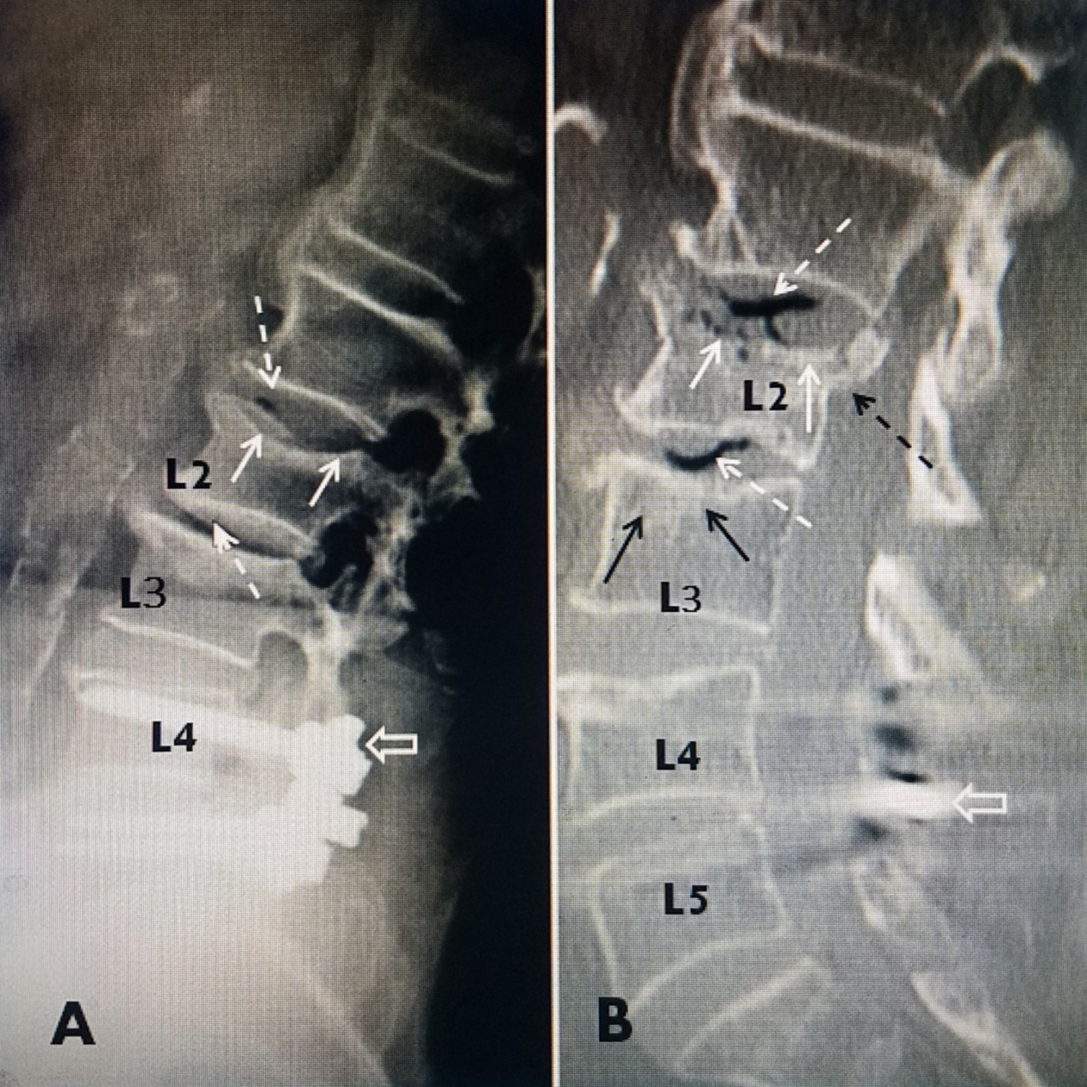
Vacuum change in the disc space surrounding an unstable fracture A: A 68-year-old female with a plain X-ray four weeks after a fall; plain radiograph showing a superior endplate fracture at L2 (solid white arrow) and previous pedicle screw fixation L4-5 (open white arrow). There is a subtle vacuum change at the L1-2 and L2-3 disc spaces (dashed white arrow). B: Because of persistent upper lumbar pain after six months conservative treatment, sagittal reconstruction of computerized tomography (CT) was performed and now clearly shows extensive vacuum changes at the L2-3 and L1-2 disc spaces (dashed white arrows). There is now a broader L2 superior endplate collapse with irregular interruptions in the continuity of the superior endplate (solid white arrows). On the CT, there is clearly the visualization of a more extensive posterior displacement of the superior endplate of L2 (dashed black arrow) into the ventral spinal canal. Part of the L4 screw is indicated by the open white arrow.
